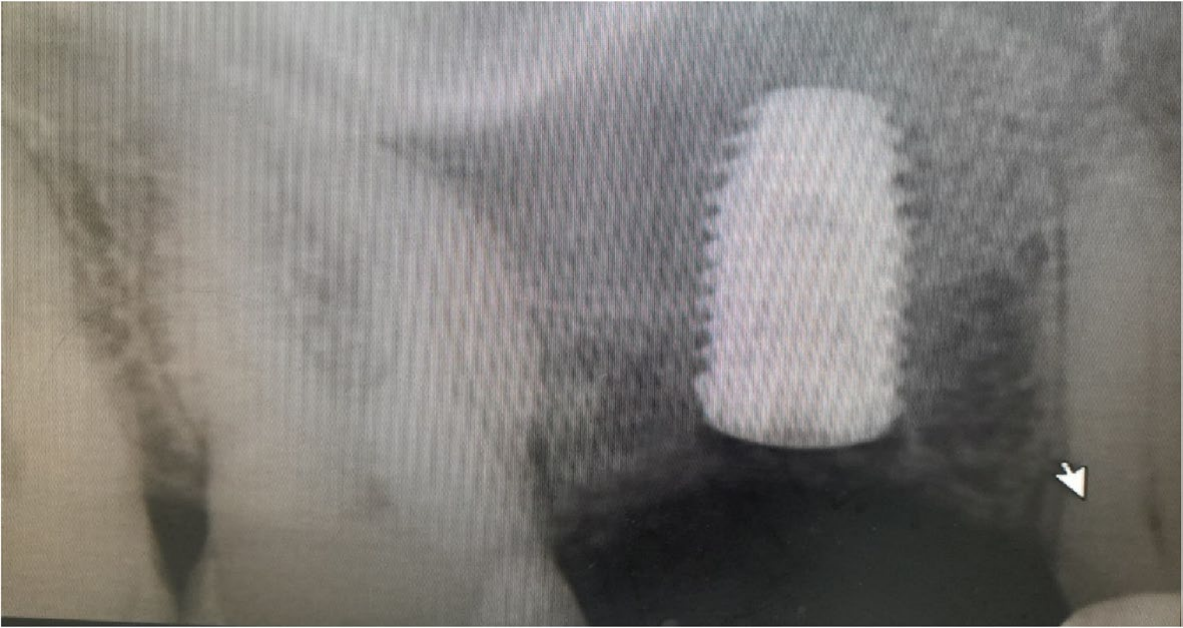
Post-operative periapical Radiograph of implant in place after sinus floor elevation
